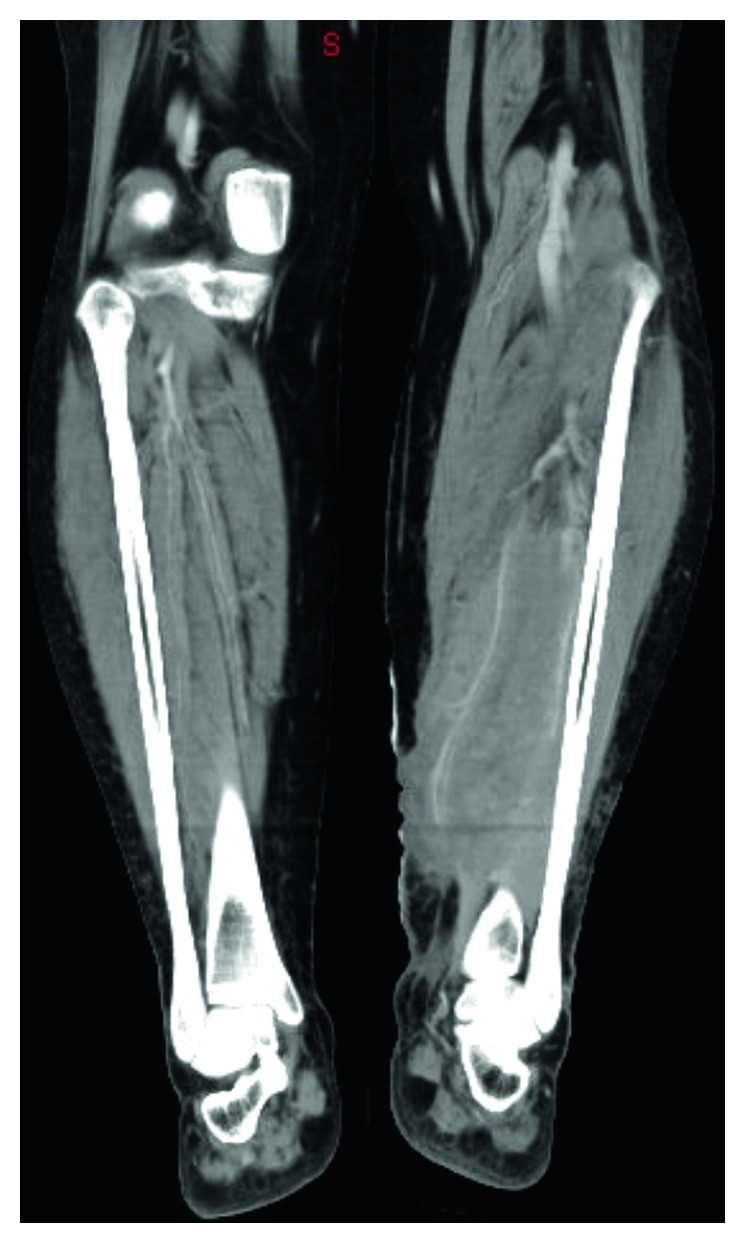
Contrast-enhanced CT of lower extremities. CT shows hypodense lesions and fluid collection in the medial aspect of the posterior compartment of the left lower leg.
